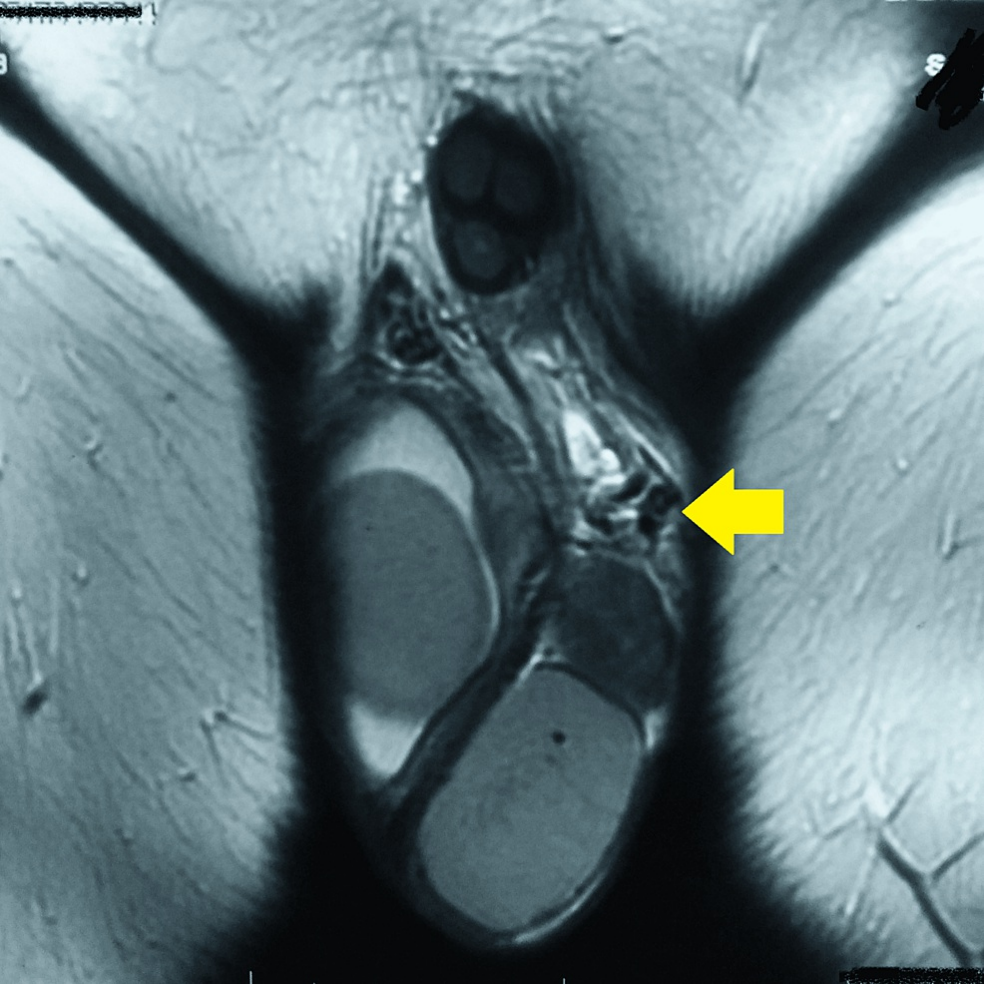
MRI of the scrotum suggestive of nodular thickening in the left epididymis MRI: Magnetic Resonance Imaging
